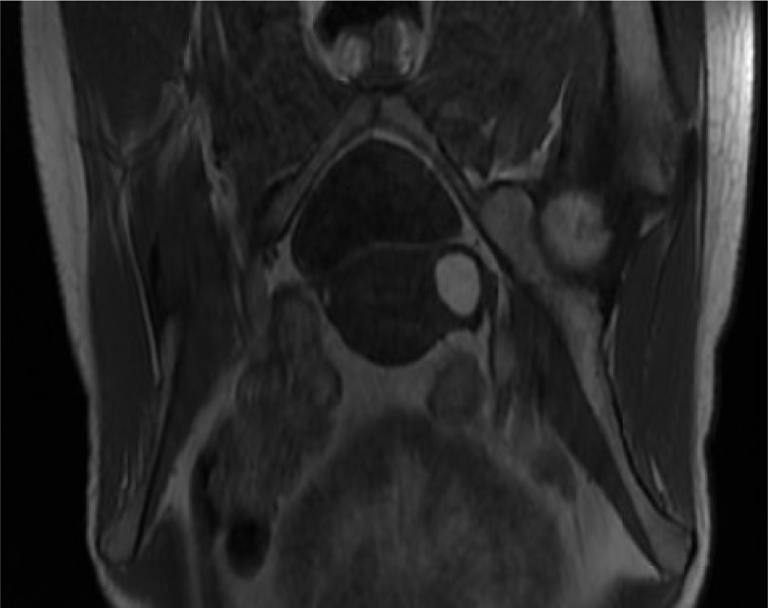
MRI with a coronal T1-weighted image of the pelvis showing a bright T1 well-circumscribed lesion in the left uterine myometrium. MRI = magnetic resonance imaging.
